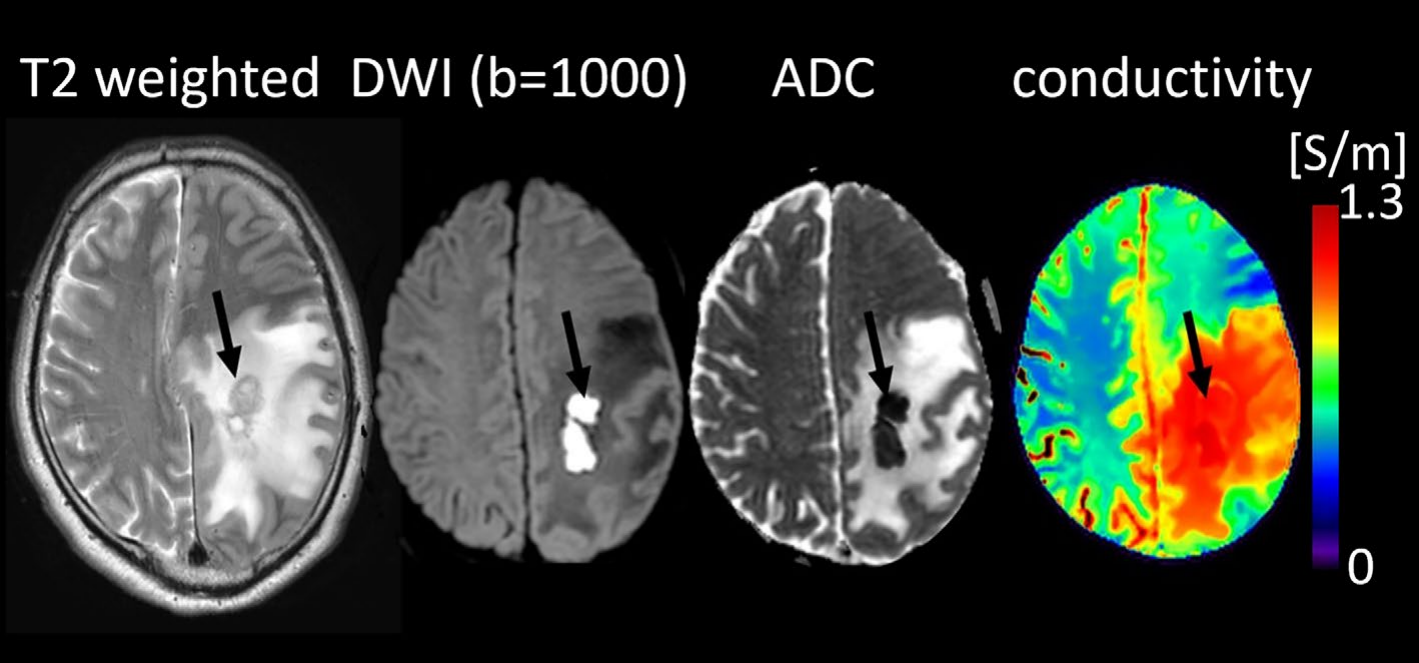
A case of cerebral abscess. T2 weighted image shows two ring‐like lesions (black arrows) and surrounding edema. DWI (*b* = 1000 s/mm^−2^) shows hyperintensity and the corresponding ADC map shows low ADC values, within the lesions—suggestive of restricted diffusion. Conductivity map shows very high conductivity within the lesions and surrounding edema.
